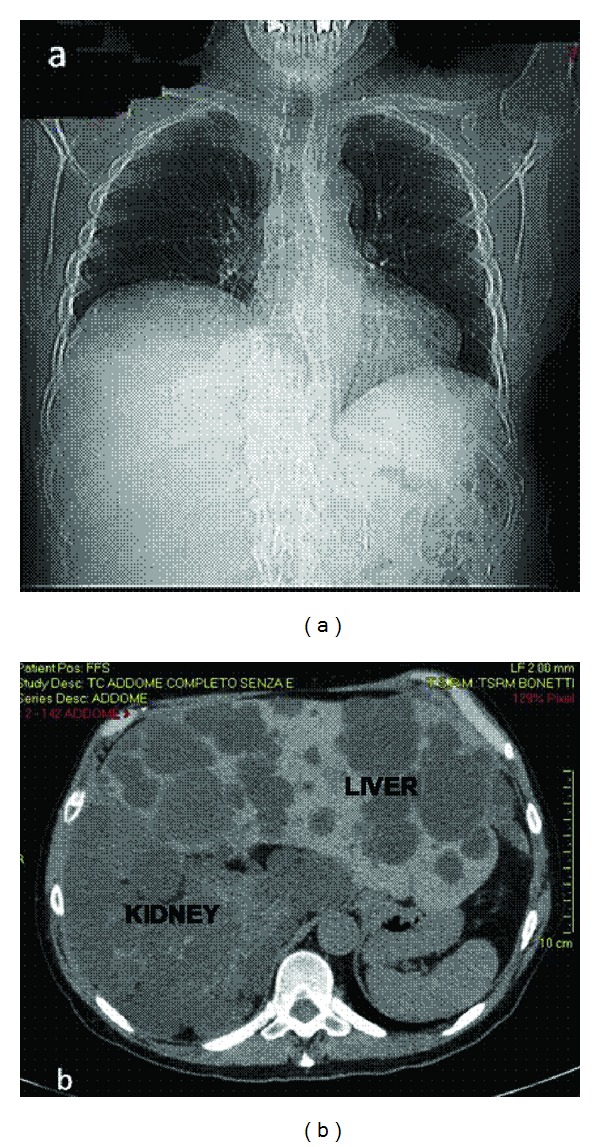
(a) Chest X-ray showing the elevation of the right diaphragm; (b) abdomen multislices CT-scan view showing the hepatic and kidney cysts.
